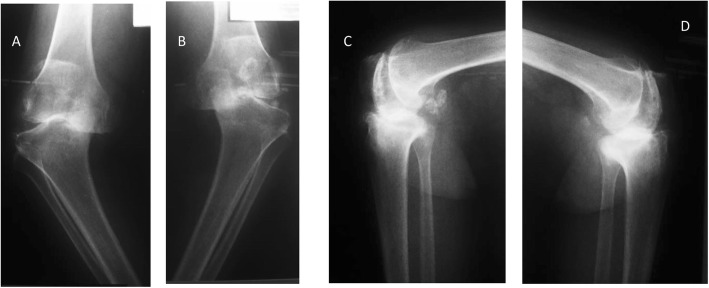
Pre-op radiographs of one of our patients who had Type 2B defects bilaterally. a Anteroposterior (AP) Standing of Right knee. b AP Standing of Left knee. c Lateral view of right knee. d Lateral view of left knee
